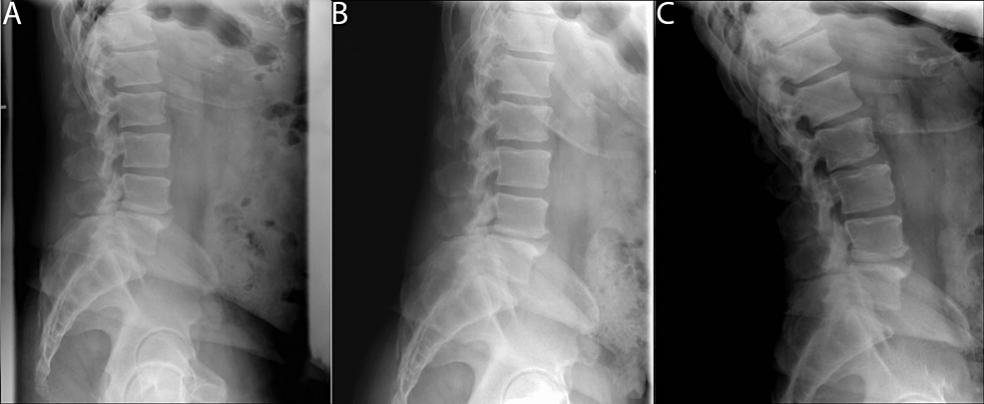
Pre-operative dynamic X-ray images of the lumbar spine showing grade 1 spondylolisthesis of L4-L5 (A) neutral (B) flexion (C) extension.
